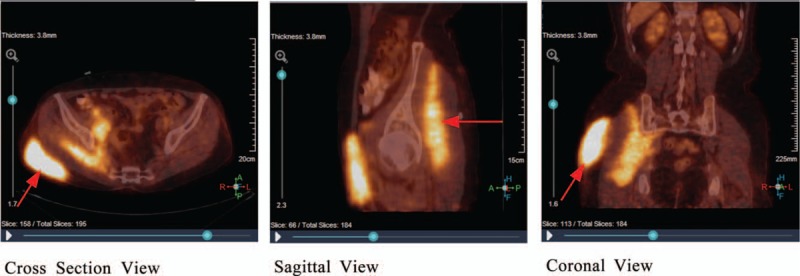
PET/CT scan of the tumor before treatment. PET/CT = positron emission tomography/computed tomography.
